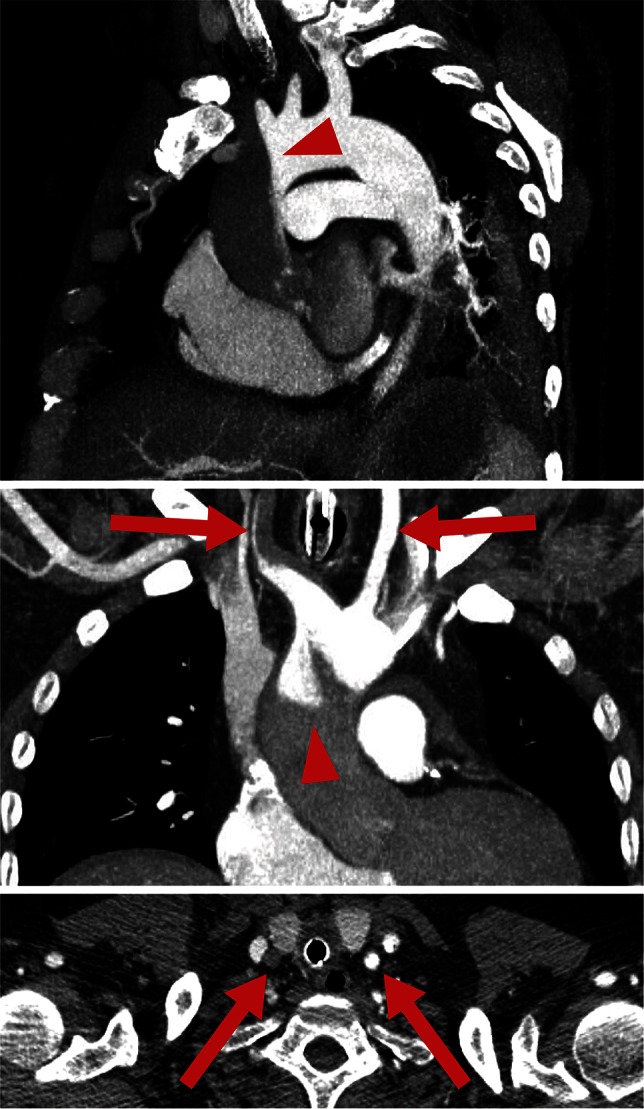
Watershed phenomenon during veno-arterial ECMO visualized by computed tomography. Antegrade blood flow (low contrast) from the heart competes with retrograde blood flow (high contrast) from the ECMO in the aorta, resulting in a watershed phenomenon (*arrowhead*). Here computed tomography of a patient with pulmonary embolism and reduced cardiac output demonstrates a rather proximal watershed, leading to perfusion of the right carotid artery with “heart blood” (*dark*) and the left carotid artery with “ECMO blood” (*bright*, *arrows*). *Upper panel* sagittal oblique maximum intensity projection (MIP), *middle panel* coronal oblique MIP, *lower panel* transverse plane
